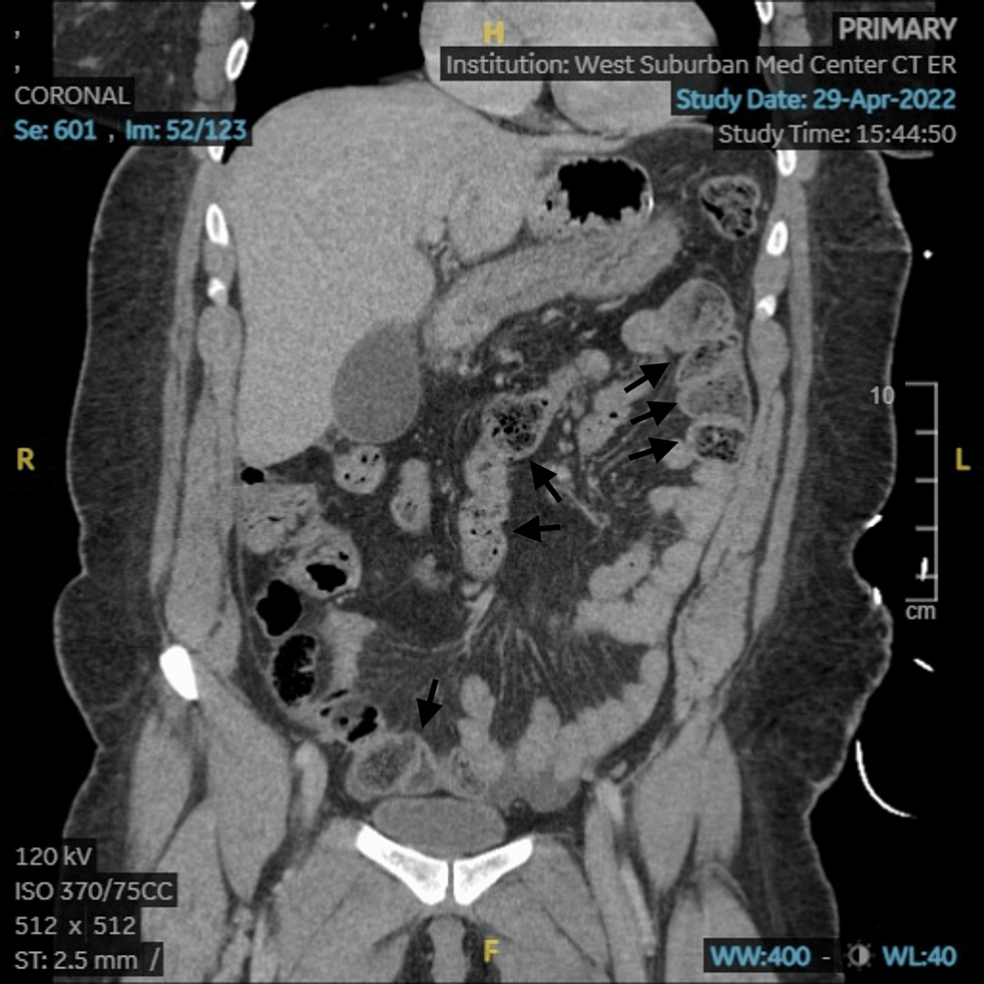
CT of the abdomen and pelvis with intravenous contrast coronal section showing mild circumferential distal small bowel wall thickening and mildly dilated loops of small bowel with feces
